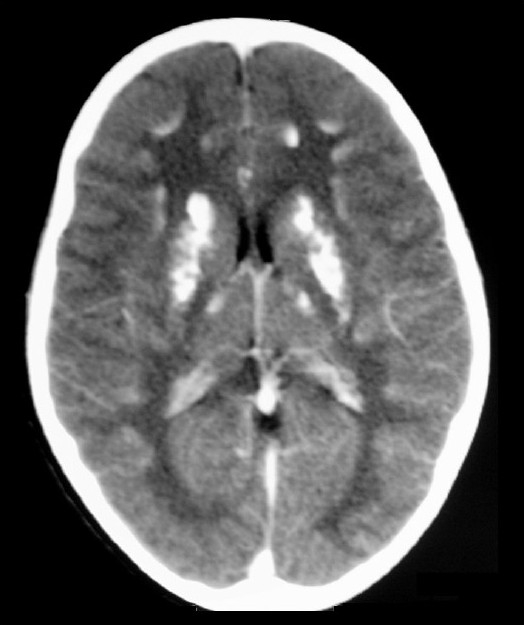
Photograph shows CT Scan of brain (plain) revealing bilateral basal ganglia calcification.
